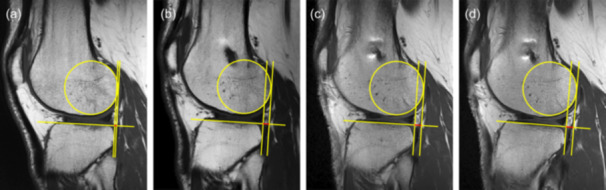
Serial magnetic resonance images demonstrating the longitudinal changes in the lateral passive anterior tibial subluxation (L‐PATS) in a representative case. Measured L‐PATS (red segments) were 1.18 mm at baseline (a), 3.67 mm at 6 months (b), 4.06 mm at 12 months (c) and 4.45 mm at 24 months (d).
